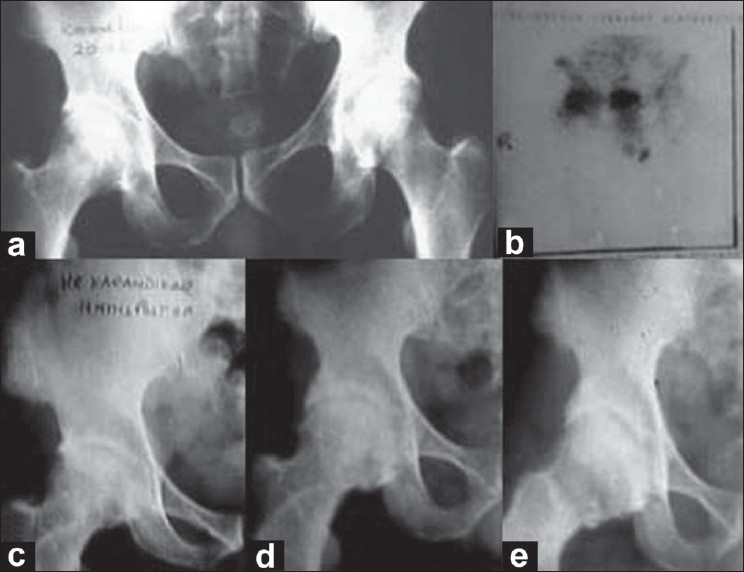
Pre-operative anteroposterior (a) X-ray of the pelvis of young male patient shows osteonecrosis of the femoral head. (b) Preoperative radionuclide bone scan showing osteonecrosis of right femoral head. (c) Immediate postoperative X-rays after vascular pedicle bone grafting. (d) Postoperative X-ray at 14 months showing good revascularization of right femoral head. (e) Postoperative X-rays at 15 years showing good revascularization of right femoral head without any collapse or arthritic changes.
